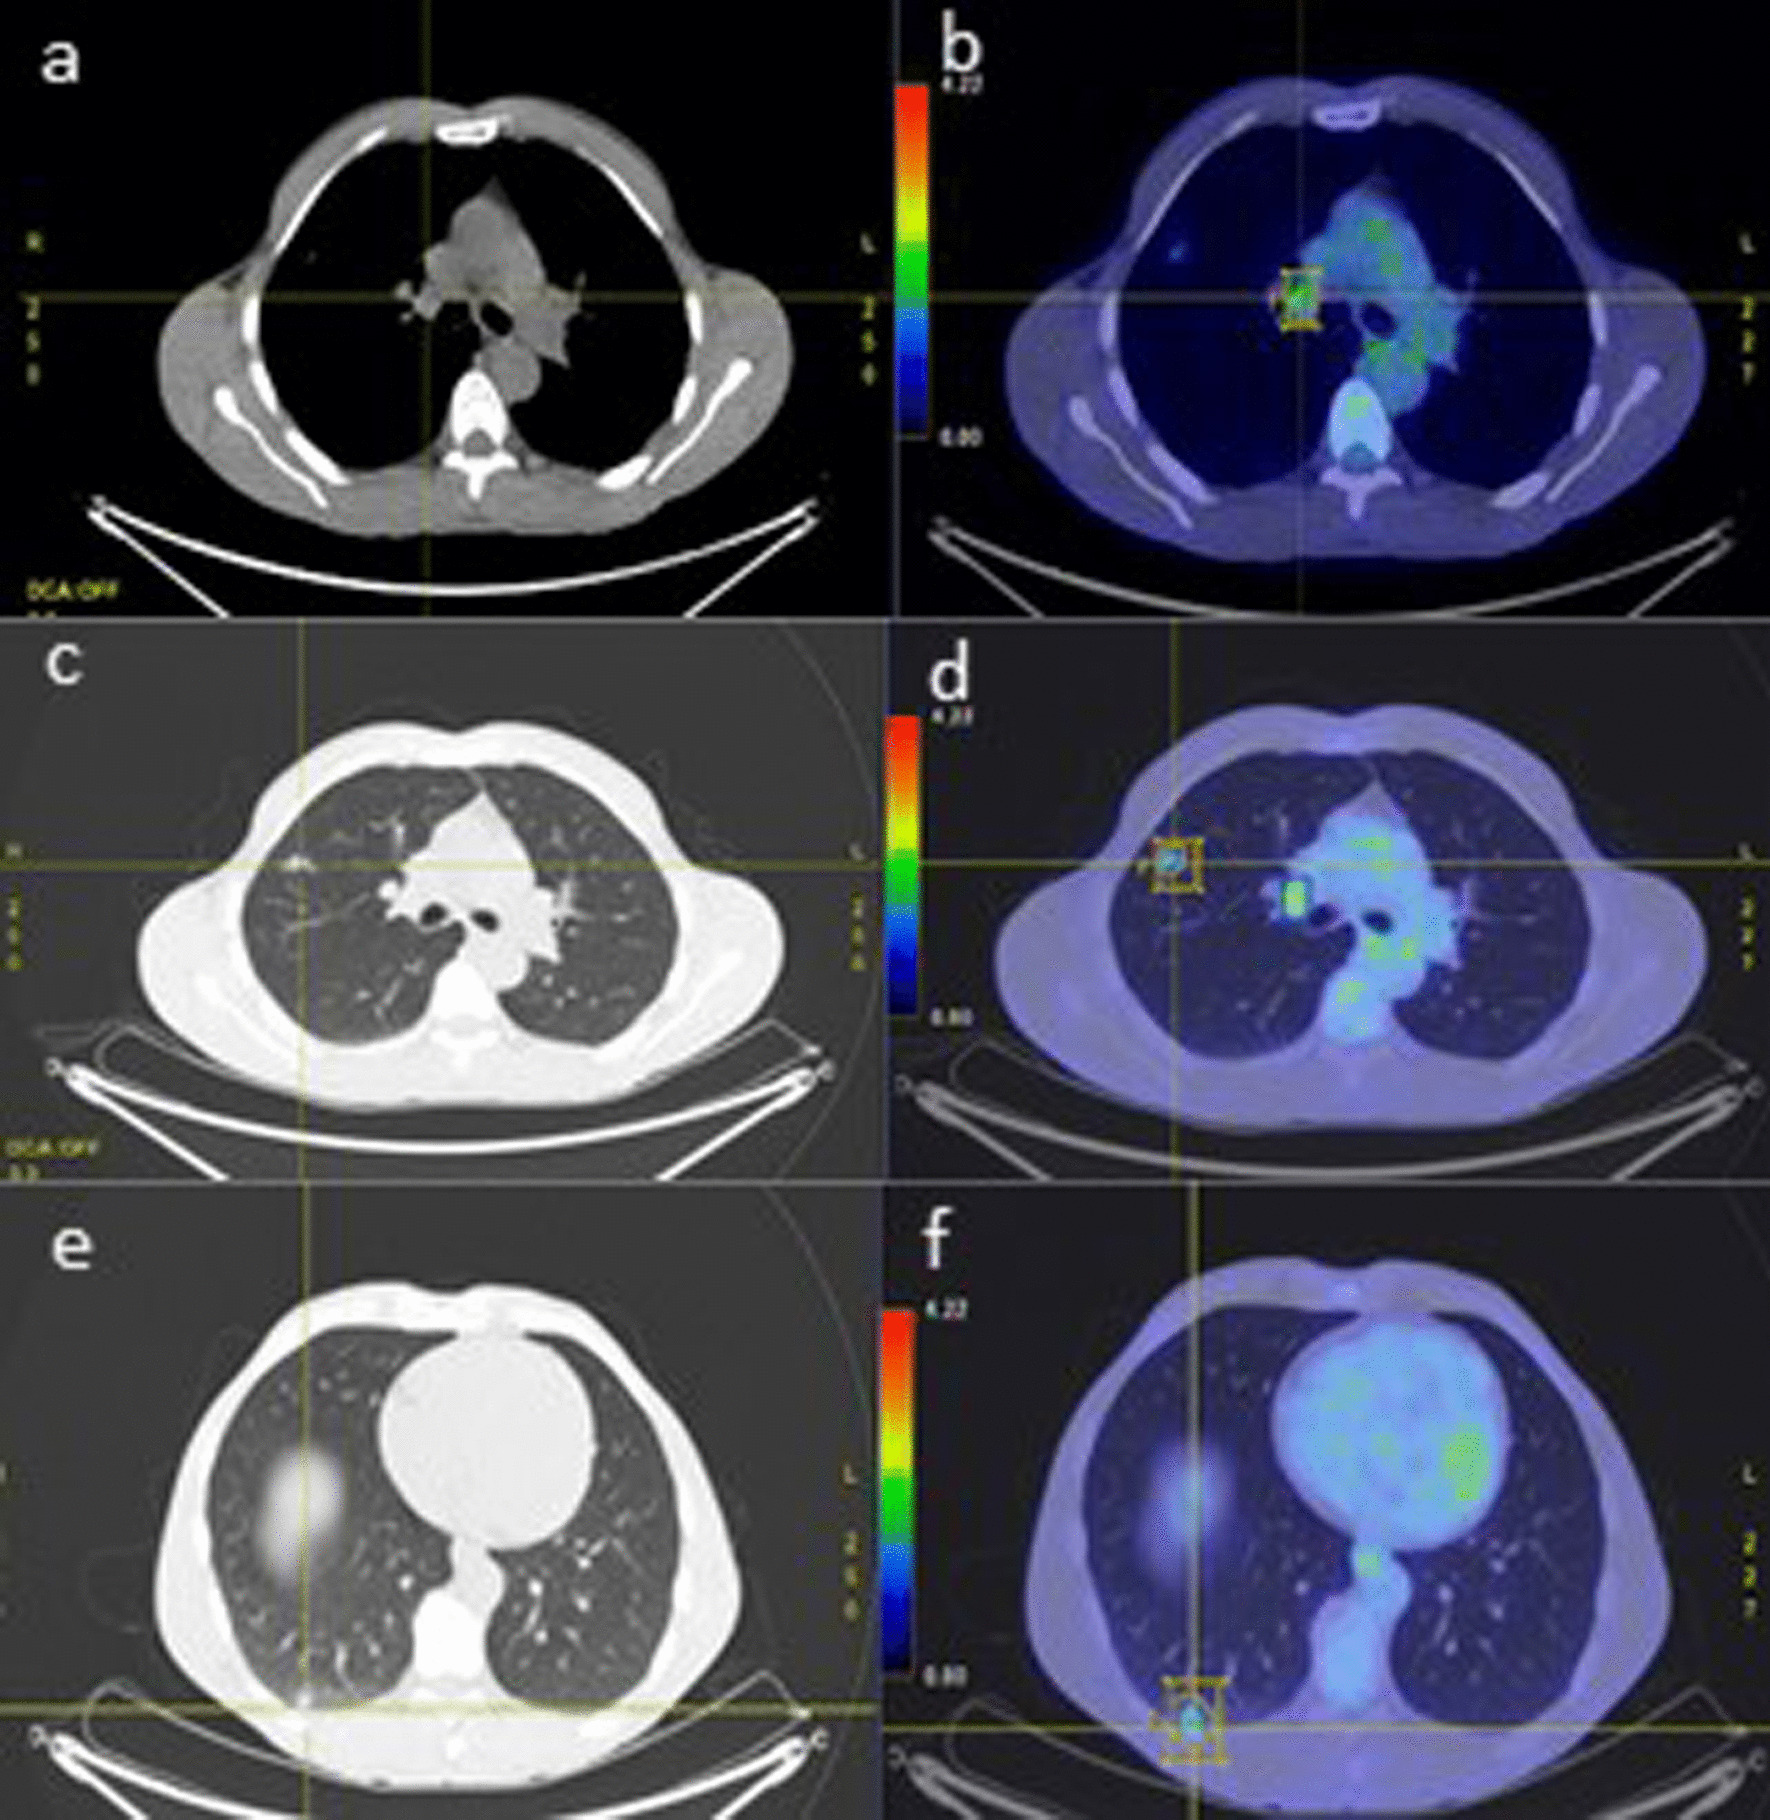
Axial positron emission tomography-computed tomography October 2019. a, b Right hilar node with equivocal fluorodeoxyglucose uptake on PET. c, d Fluorodeoxyglucose avid right upper lobe nodule. e, f Fluorodeoxyglucose avid mass in the right lower lobe
